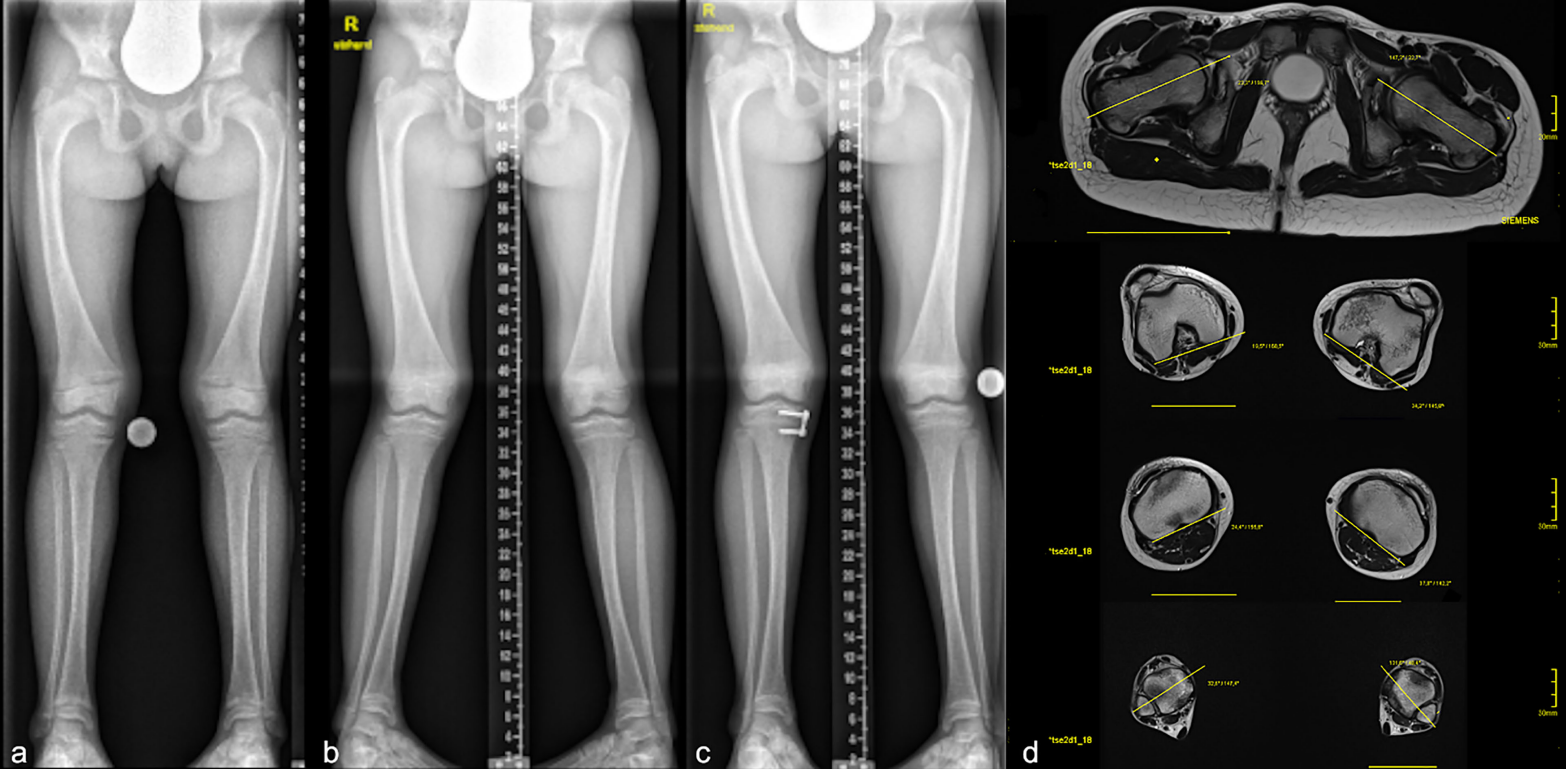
Case 2: Radiographs (A-C) and torsional MRI (D) of a 10-year-old girl undergoing Burosumab therapy. Full long leg standing radiographs were obtained prior (A) to Burosumab initiation and during treatment. Lateralization of MAD in one leg was observed at the 10-month follow-up (B). Guided growth procedure using temporary hemiepiphysiodesis was performed on the right tibia with short term success 6 months postoperative (plate not yet removed) (C). Torsional MRI showed 2° internal torsion of the femur and 3° external torsion of the tibia (D).
